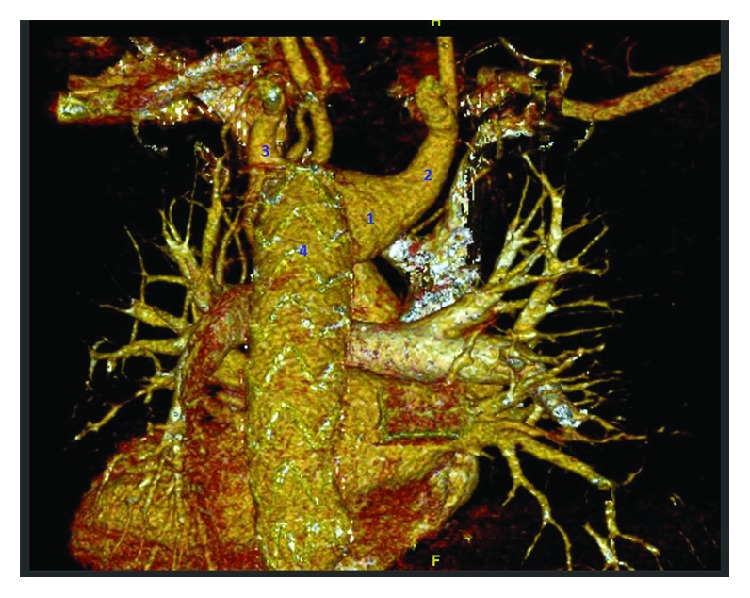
Postoperative CT aorta 3D reconstruction posterior view showing Kommerell's diverticulum (1), patent right aberrant subclavian artery (2), left subclavian artery (3), and a stent inside the descending aorta (4).
